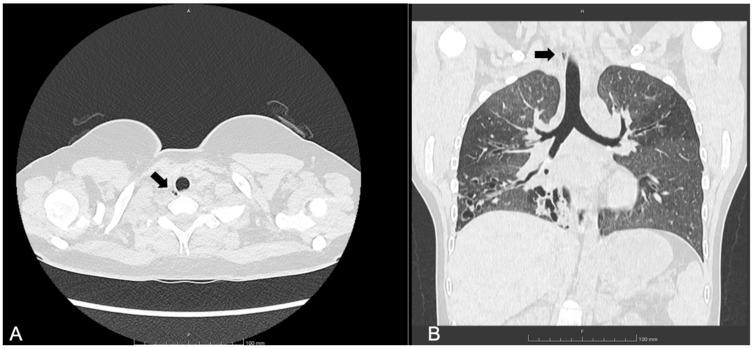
(A) Axial and (B) coronal lung windows at chest CT showing the tracheal diverticulum at the right posterolateral wall of the trachea in a cystic fibrosis patient (Patient 2). Black arrows show the tracheal diverticulum.
